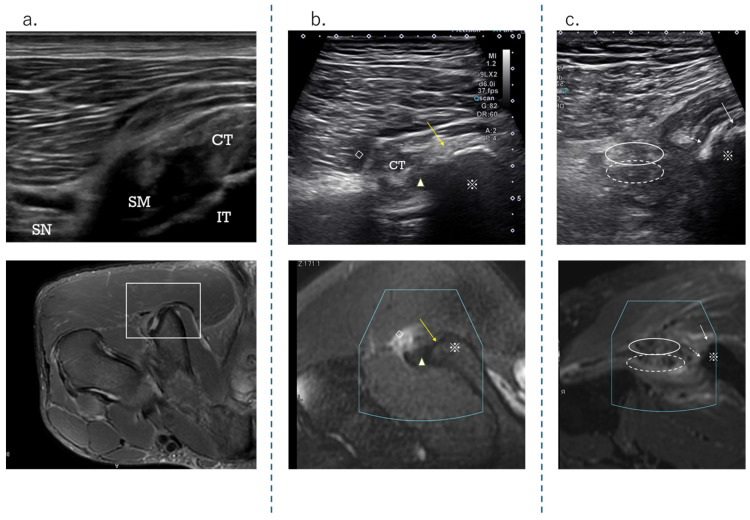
The US findings (upper row) and MRI images (lower row) of the originate tendon detachment at ischial tuberosity. (a) Detached image of the SM tendon attachment; (b) detached image of the CT attachment (yellow arrow), hematoma (white square), and SM attachment (solid white triangle); (c) detached image of the CT attachment (white arrow) and CT (white ellipse), detached SM attachment (white dashed arrow), and SMT (white dashed ellipse).
